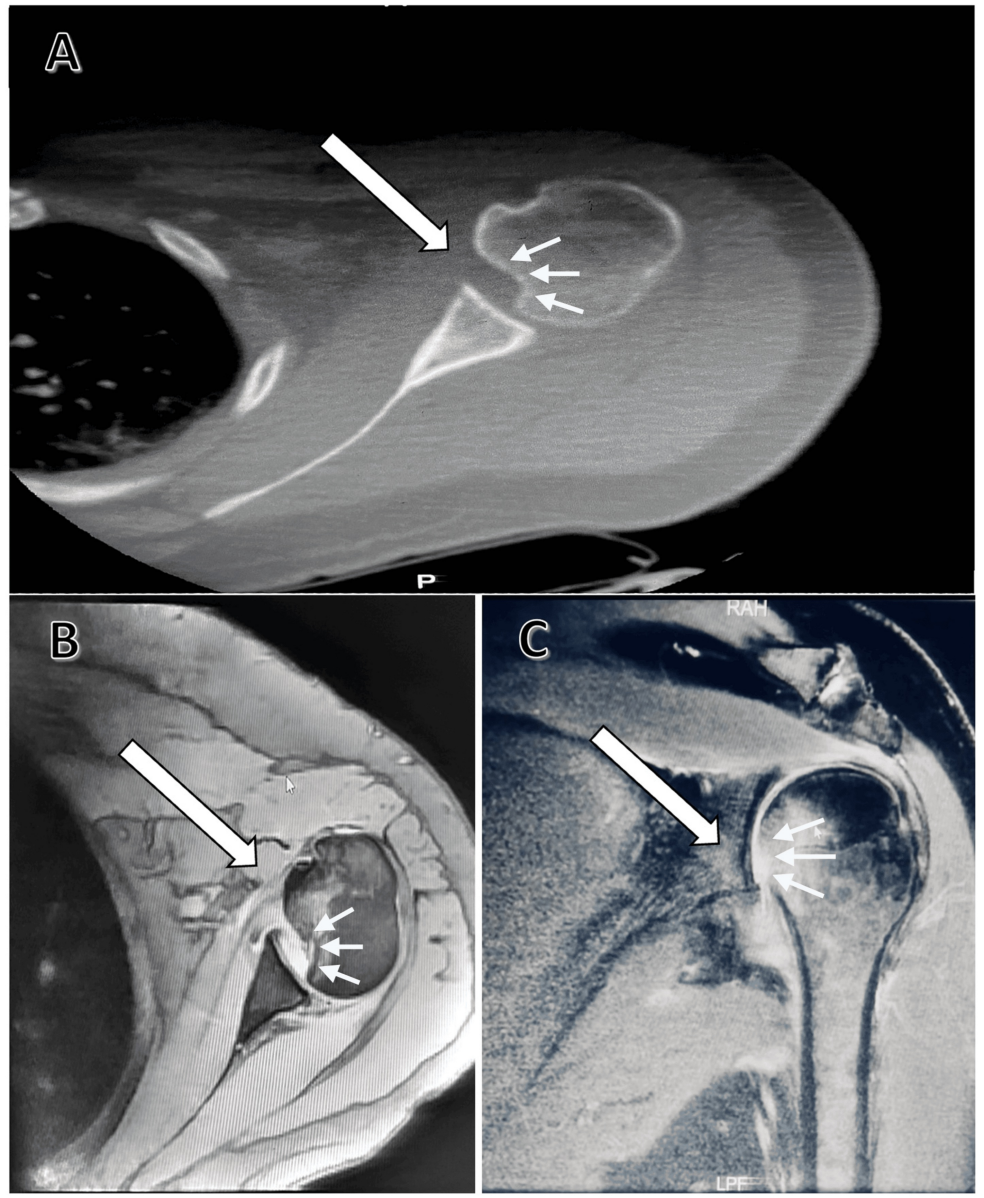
(A) CT scan of the left shoulder showing a reverse Hill-Sachs lesion affecting 25% of the humeral head. (B, C) MRI of the left shoulder showing the extent of the reverse Hill-Sachs lesion.
